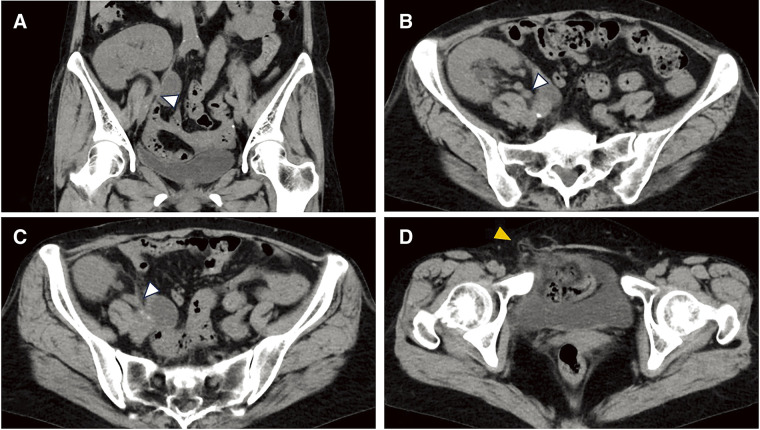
Plain CT findings. (A) Coronal view and (B–D) axial view: The ureter (white arrowhead) runs medially, dorsally, and caudally from the transplanted kidney, but its entry into the bladder is unclear. A direct hernia with fat content is observed (orange arrowhead).
